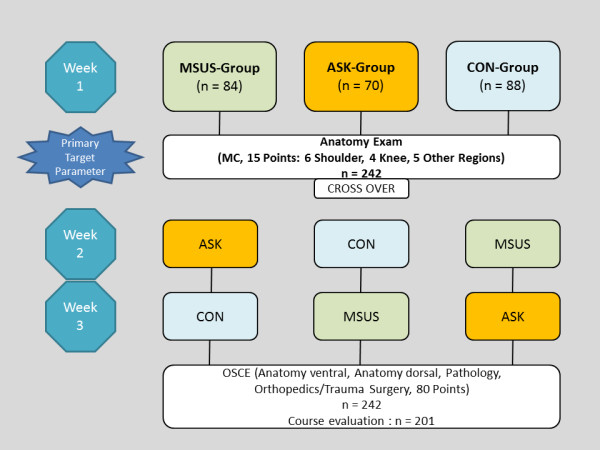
The flow of participants through each stage of the trial is shown. ASK: arthroscopy, MSUS: musculoskeletal ultrasound, CON: control-group without additional intervention (only cadaver dissection course), OSCE: objective structured clinical examination.
